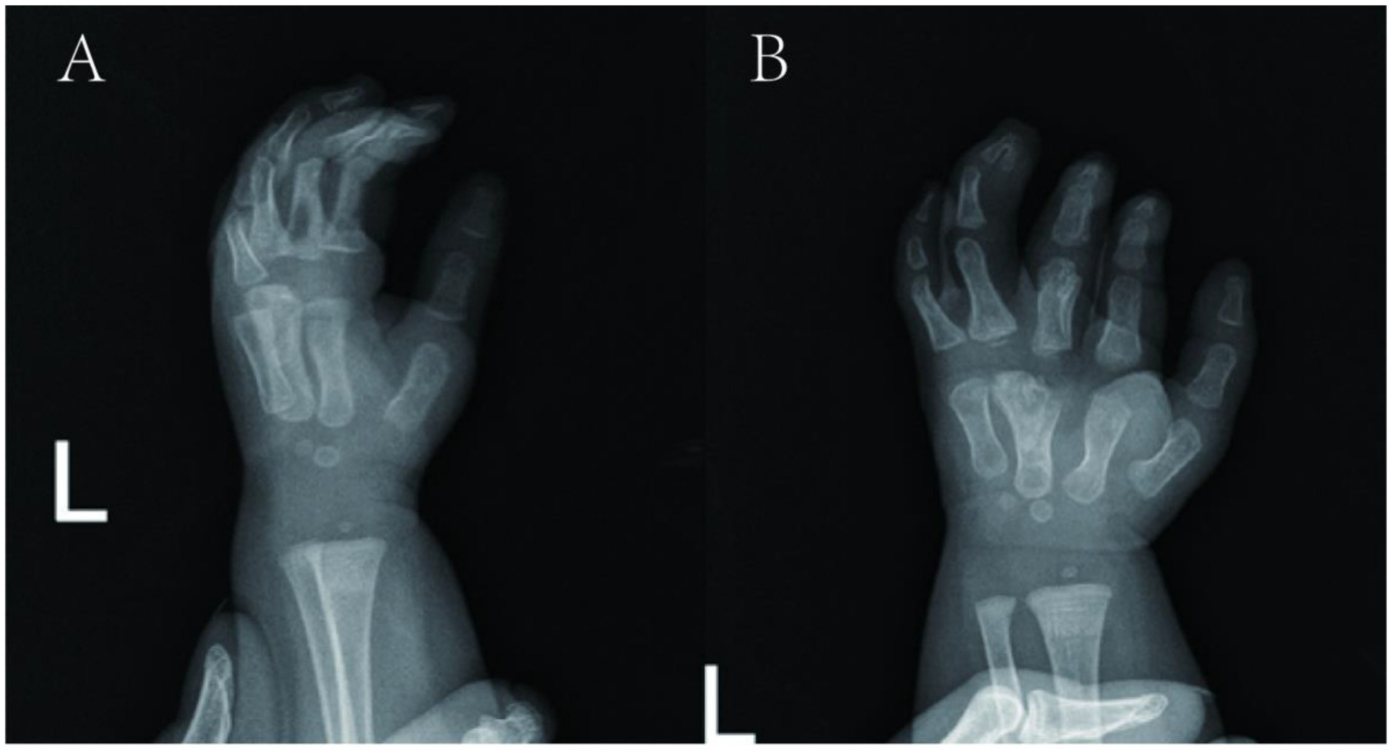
Follow-up pictures at ten weeks after the operation. (A,B) x-ray films.
